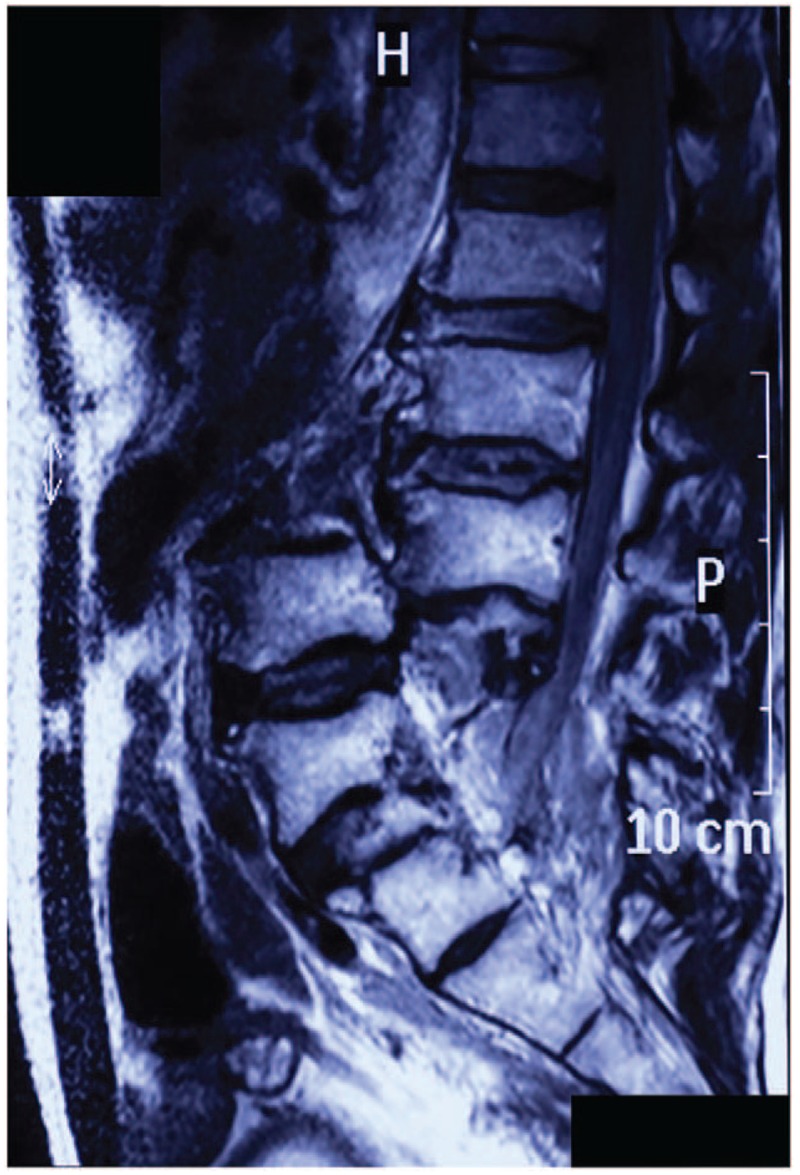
T2-weighted sagittal magnetic resonance imaging shows the neural arches at injured segments are barely damaged and the dura sac at corresponding segments is mildly compressed.
